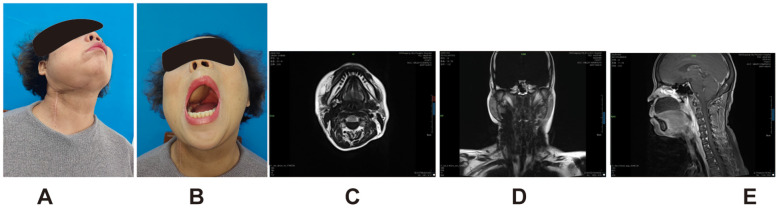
The patient’s follow-up six months after the surgery and the MRI imaging results of the patient 6 months after the surgery. (A). Postoperative view of the neck, (B). Postoperative view of the oral cavity, (C). Postoperative axial MRI, (D). Postoperative coronal MRI, (E). Postoperative sagittal MRI.
